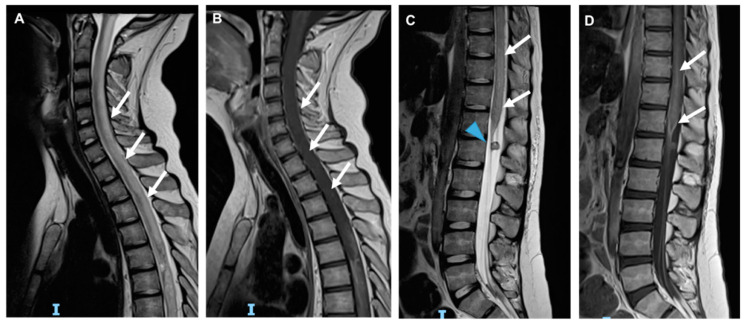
Acute myelitis due to chemotherapy (Nelarabina) in an ALL—T patient. Sagittal MRI shows the abnormal medullar signal of the spine (arrows, A) without contrast enhancement after gadolinium administration (arrows, B). (C,D) show an abnormal hyperintensity of the distal part of the medullar signal (arrows, C) associated with a peripherical aspecific gadolinium enhancement (arrows, D). A metastatic nodule was also seen (arrowhead, C).
